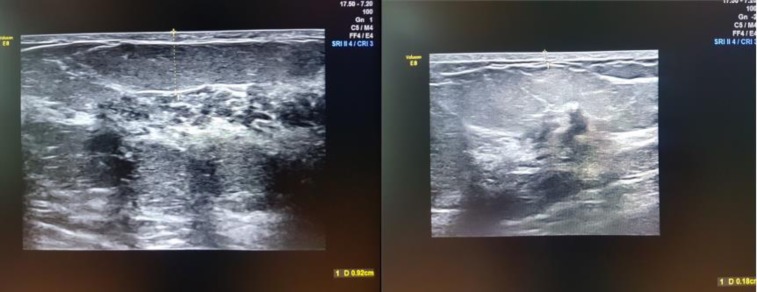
Preoperative breast ultrasound – subcutaneous tissue measurement and skin thickness in the established points.
